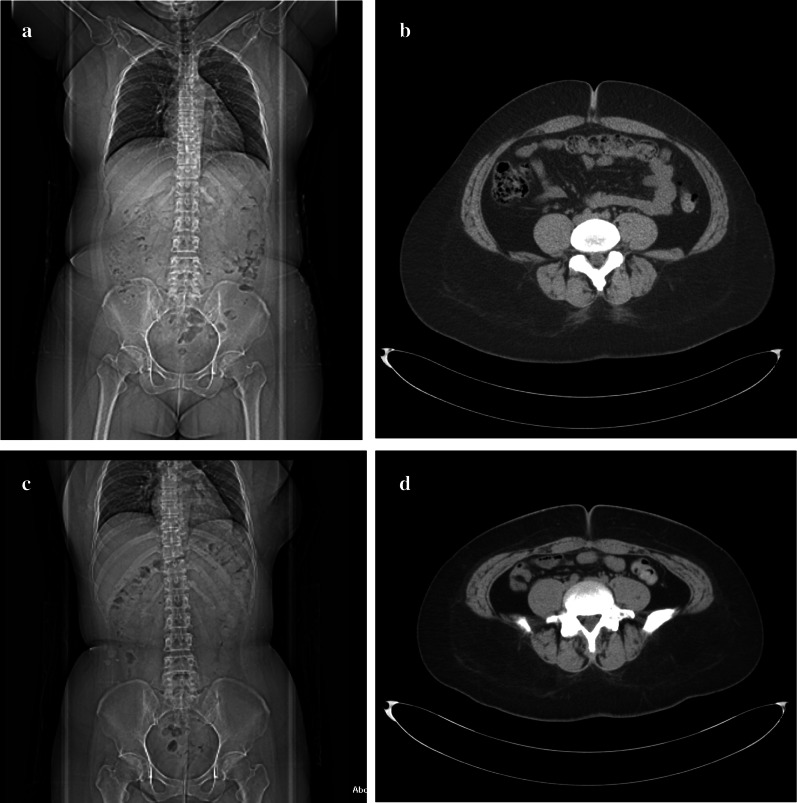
Computed tomography images. a Overall image before surgery, showing b a preoperative visceral fat area of 162.6 cm^2^ and subcutaneous fat area of 527.9 cm^2^. c Overall image, 1-year after the surgical procedure, showing a decrease in d the visceral fat area to 44.8 cm^2^ and the subcutaneous fat area to 408.8 cm^2^
